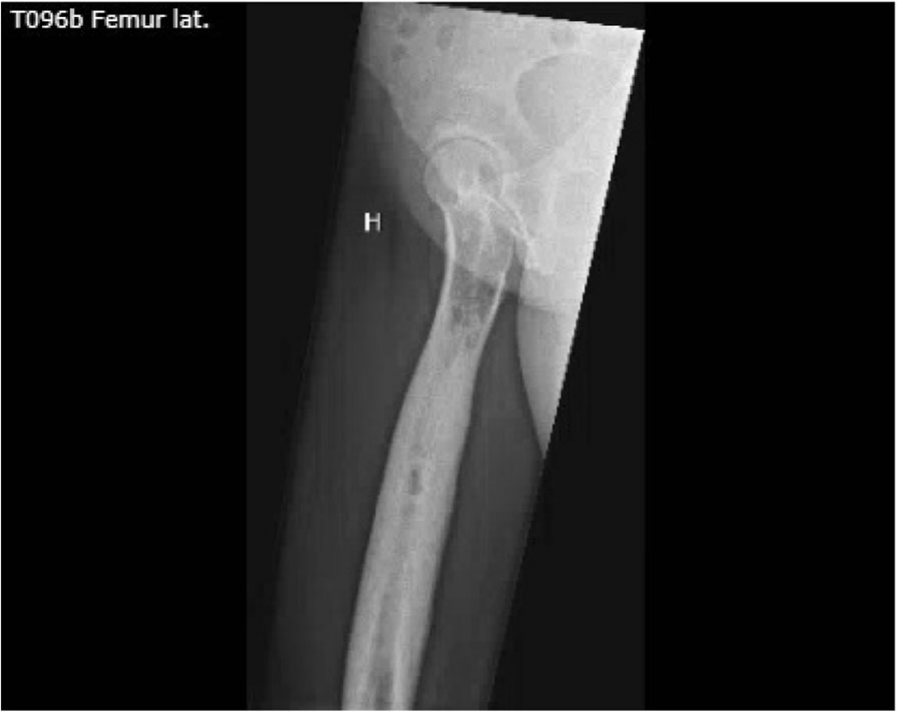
X-ray right femoral shaft. X-ray of the patient’s right femoral shaft showing irregular hyperostosis
